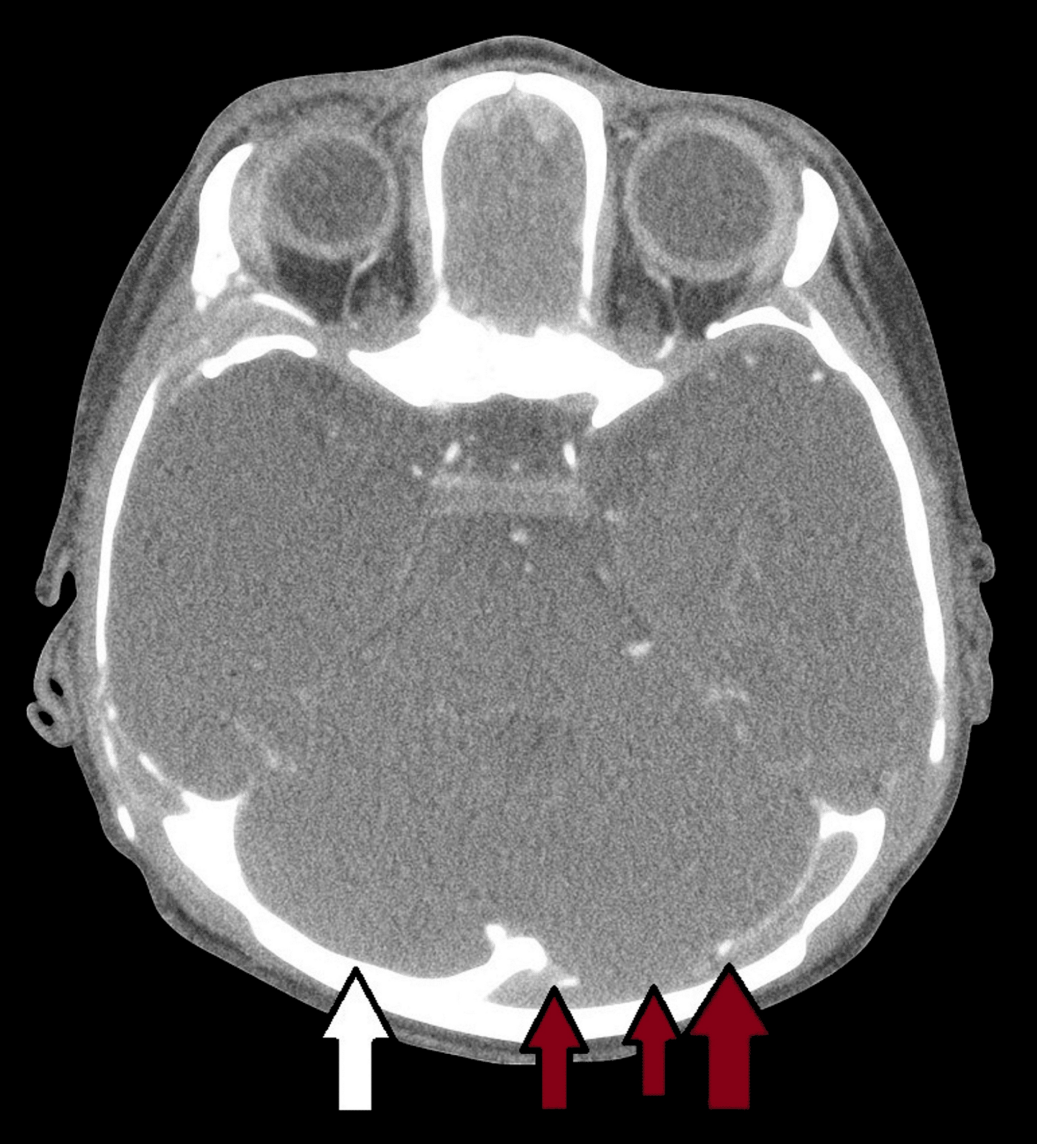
Brain CT with contrast showing partial central venous sinus thrombosis involving the superior sagittal and the left transverse sinus Red arrow showing filling defect within the posterior distal superior sagittal sinus with extension into the proximal left transverse sinus, representing a tiny residual thrombus. White arrow showing normal right transverse sinus pointed for comparison.
